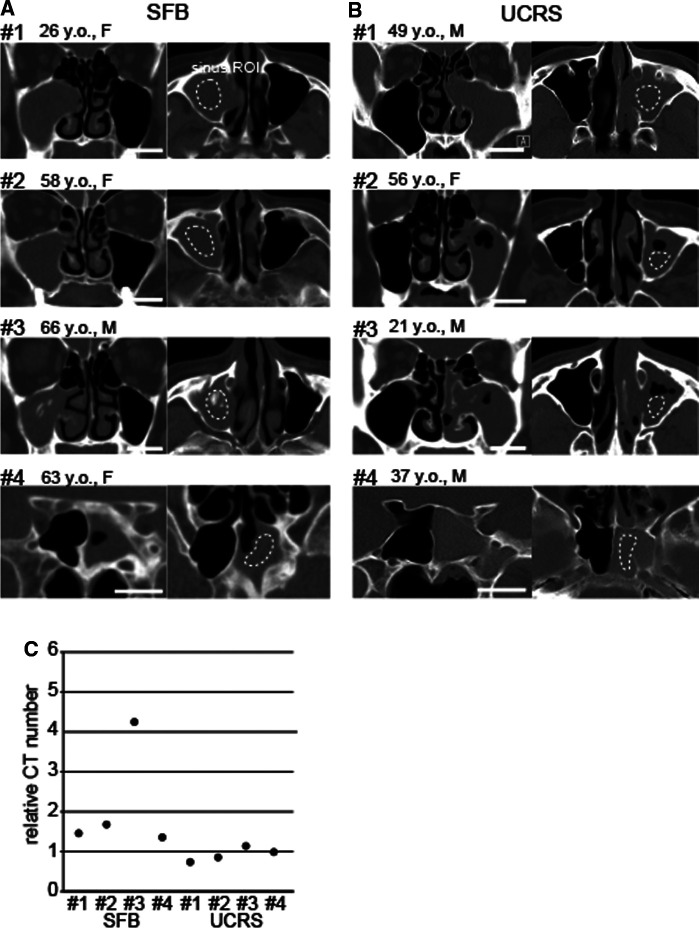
Representative images of sinus fungal ball (SFB) and unilateral nonfungal chronic sinusitis (UCRS), (A) CT images of SFB cases (#1–#4). ROIs were defined in the affected sinus (dotted circle), and the brainstem was used as a control area. ROI, region of interest. Scale bar, 20 mm. (B) CT images of UCRS cases (#1–#4). ROIs were defined in the affected sinus (dotted circle), and the brainstem was used as a control area. Scale bar, 20 mm. (C) Relative CT numbers in SFB and UCRS cases. Each dot represents the relative CT number of an individual case.
